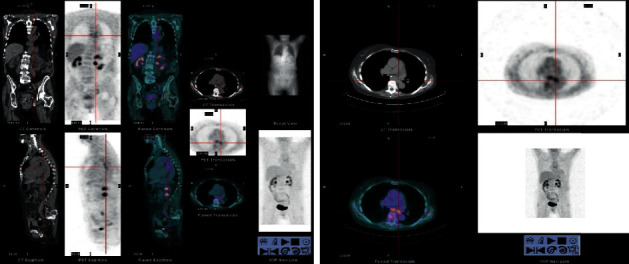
PET-CT showing an already-known metabolically active esophageal lesion, with possible adjacent ganglion metastasis.
